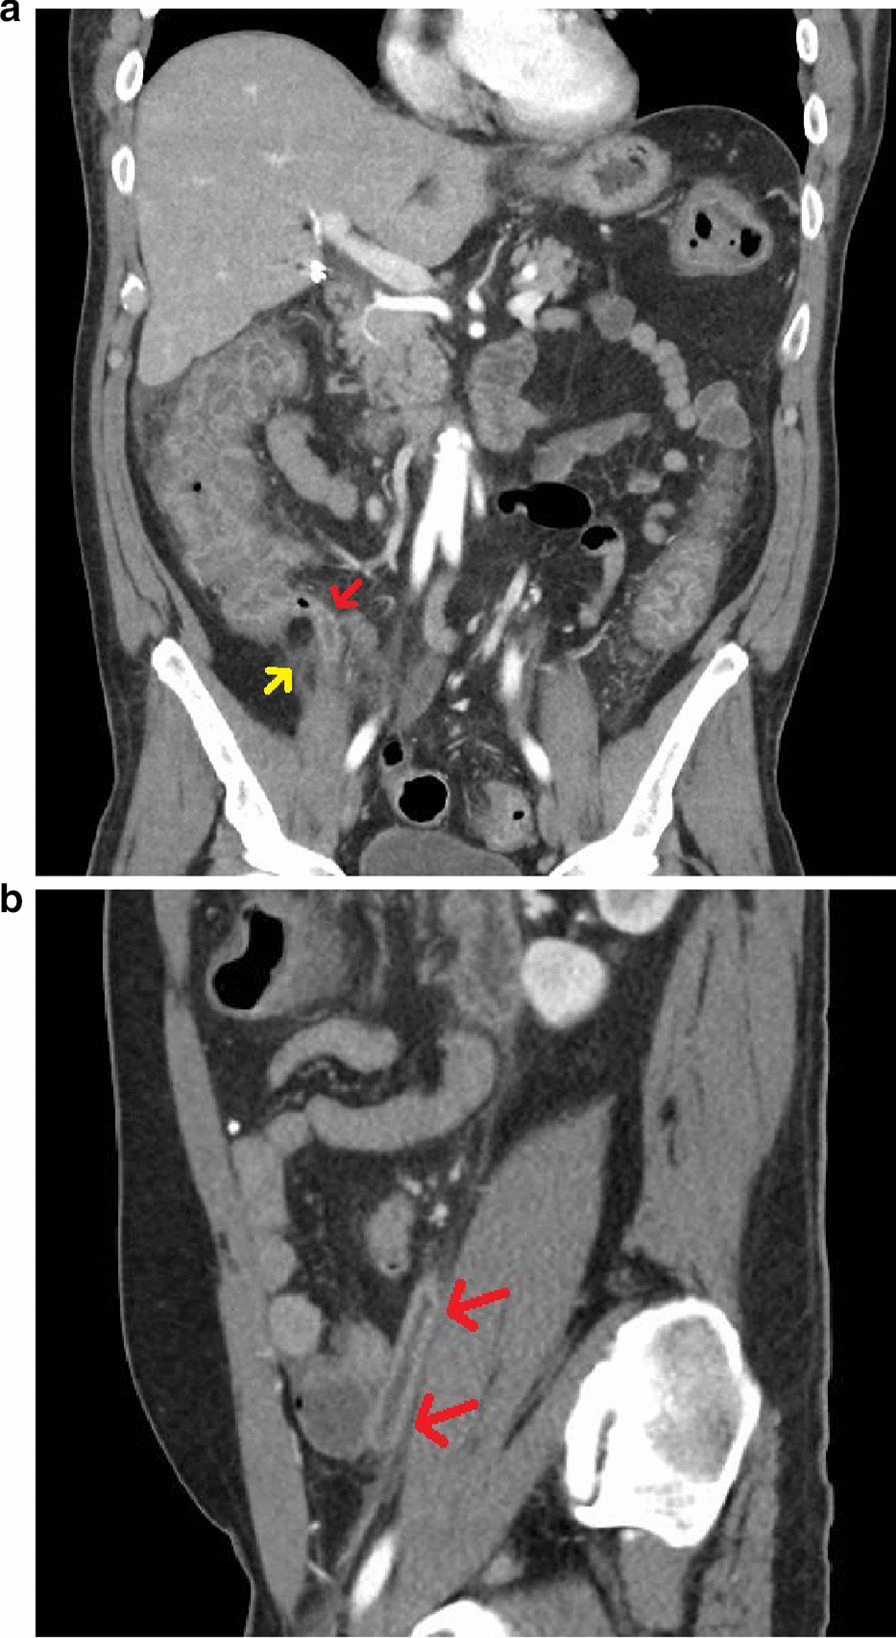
Coronal (a) and sagittal (b) CT of the abdomen and pelvis with contrast demonstrating enlargement of the appendix with wall thickening and enhancement (red arrows). Periappendiceal inflammation is also present (yellow arrow)
